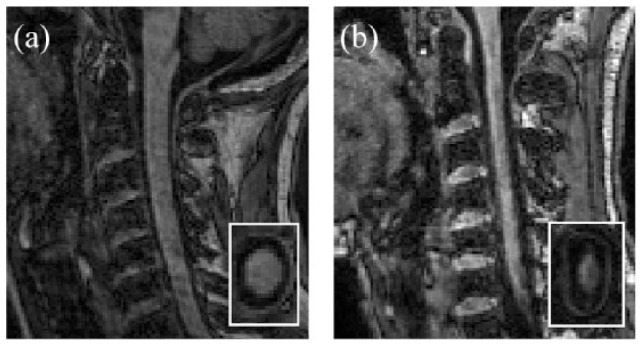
Spinal cord atrophy visible on conventional MRI. Cervical cord MRI with sagittal and C2 axial (inset, used for spinal cord cross-sectional area measurements) views in CIS (a) and PPMS (b). CIS, clinically isolated syndrome; MRI, magnetic resonance imaging; PPMS, primary progressive multiple sclerosis.
